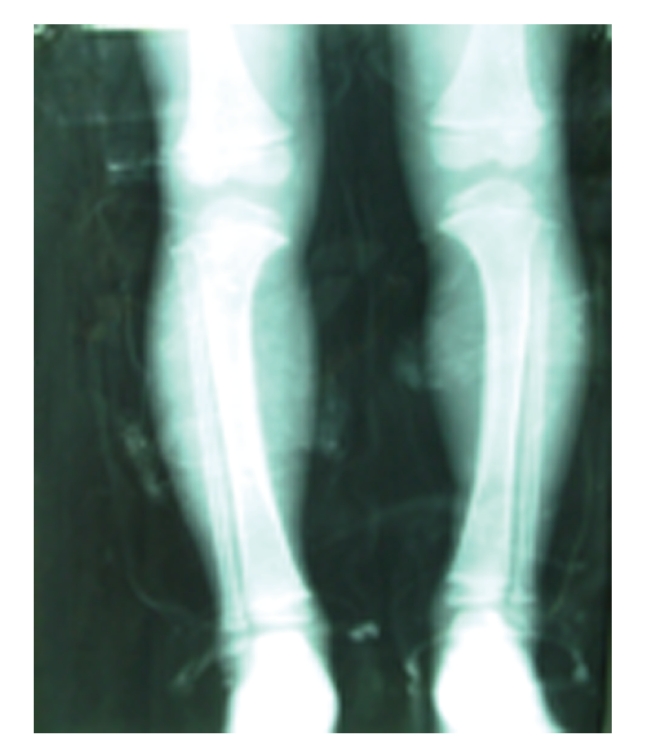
X-ray for a 7-year old boy.
